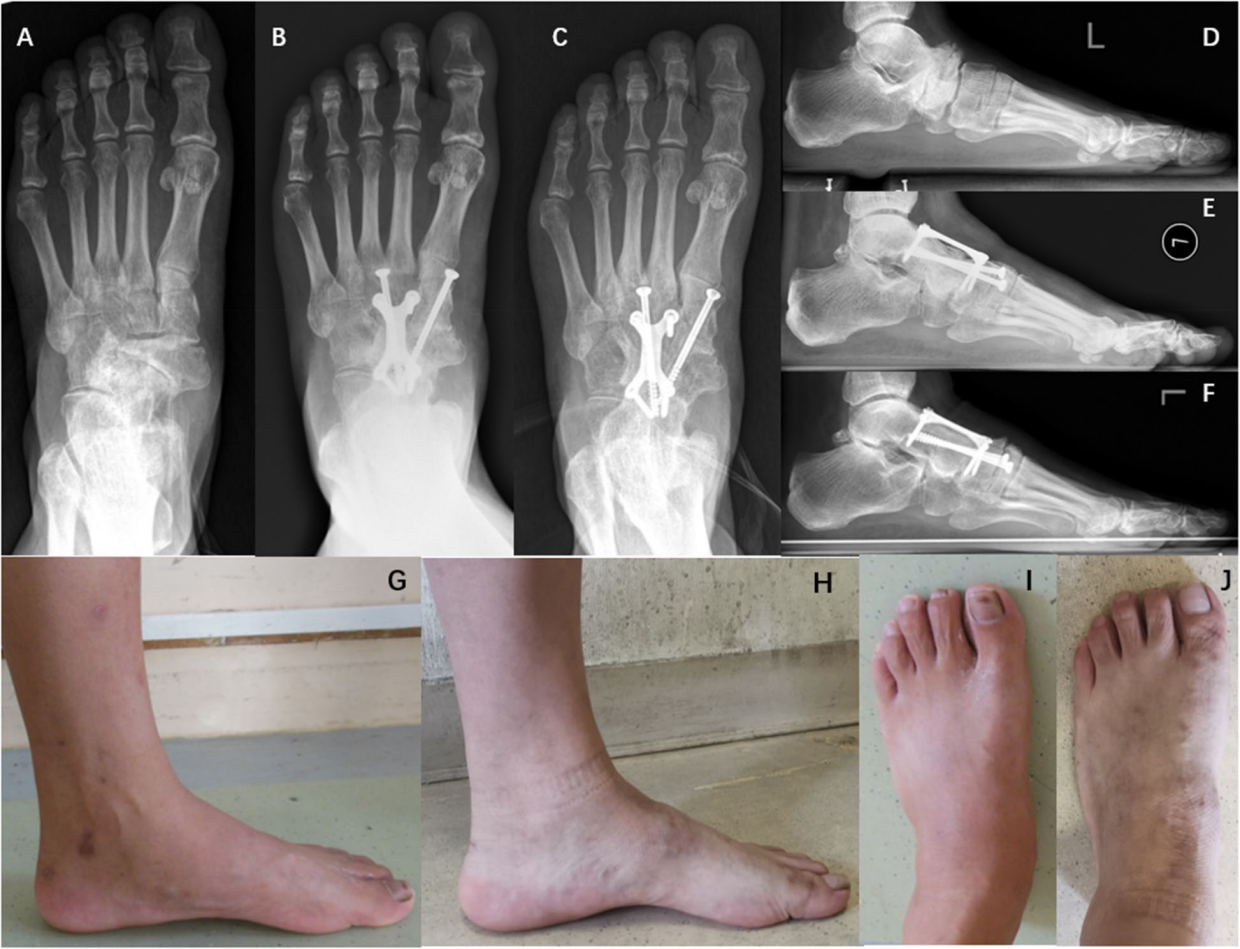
Patient No. 11. A-C Weight-bearing AP X-ray films before the operation, 3 months after the operation and last follow-up. Improved forefoot abduction, no nonunion and bone resorption; D-F Weight-bearing lateral X-ray films before the operation, 3 months after the operation and last follow-up. And lateral Meary's angle backs to normal; G-J Appearance before operation and last follow-up
